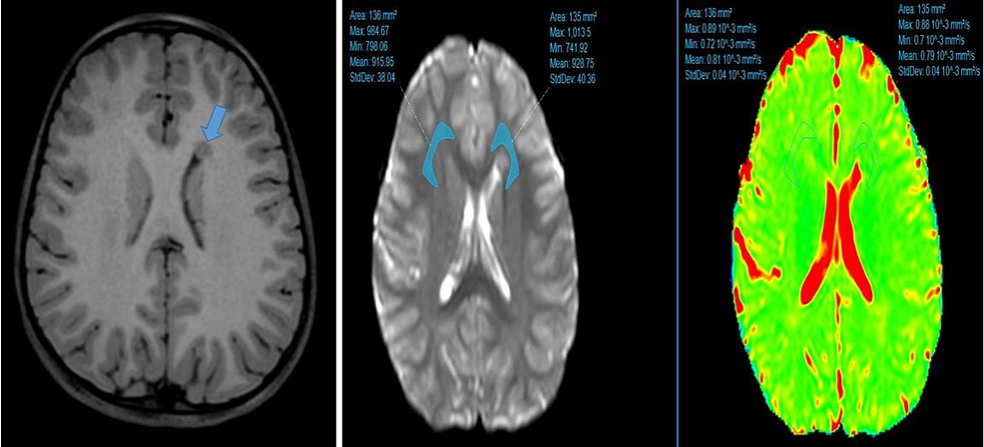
Axial T1-weighted MRI showed subependymal heterotopia in the left frontal DWI showed ADC measurement from the white matter around the heterotopia focus and from the opposite cerebral hemisphere. DWI: diffusion-weighted imaging; ADC: apparent diffusion coefficient
